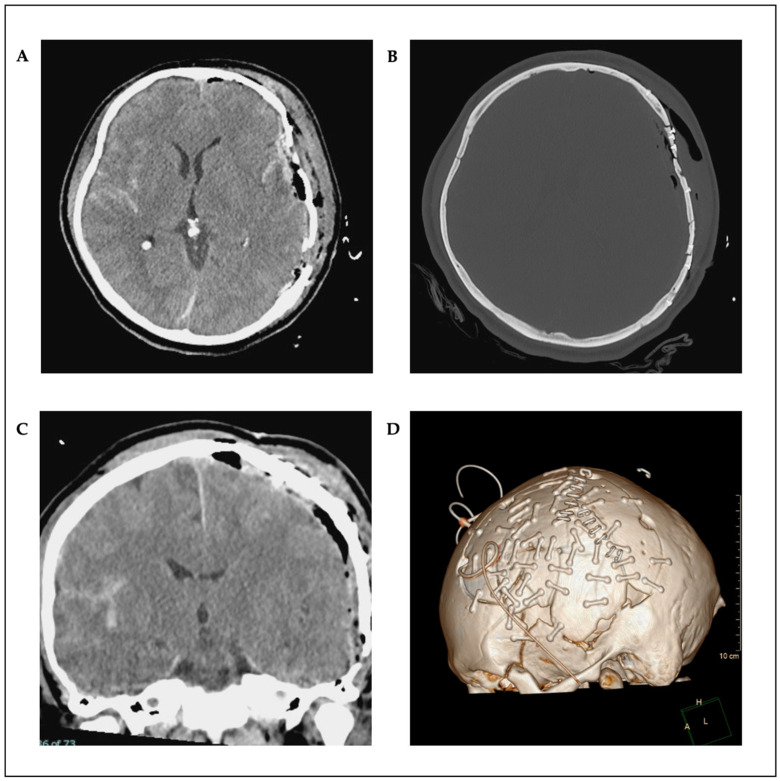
Postoperative axial (A,B) and coronal (C) CT scans; as well as 3D reconstruction (D).
